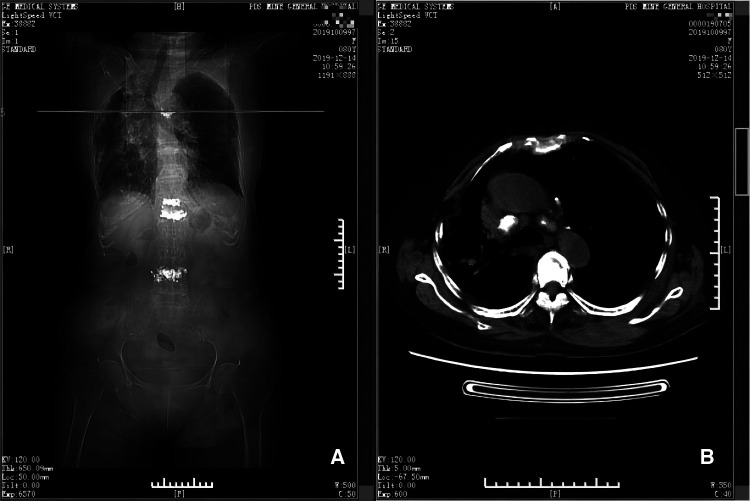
CT scan taken on 12th day postoperatively (panels A and B). Panel A depicts the position (horizontal line) of the CT scan shown in B, at the level of the 5th thoracic vertebra. Panel B shows there is little remaining hematoma near the 5th thoracic vertebra and adjacent ribs.
